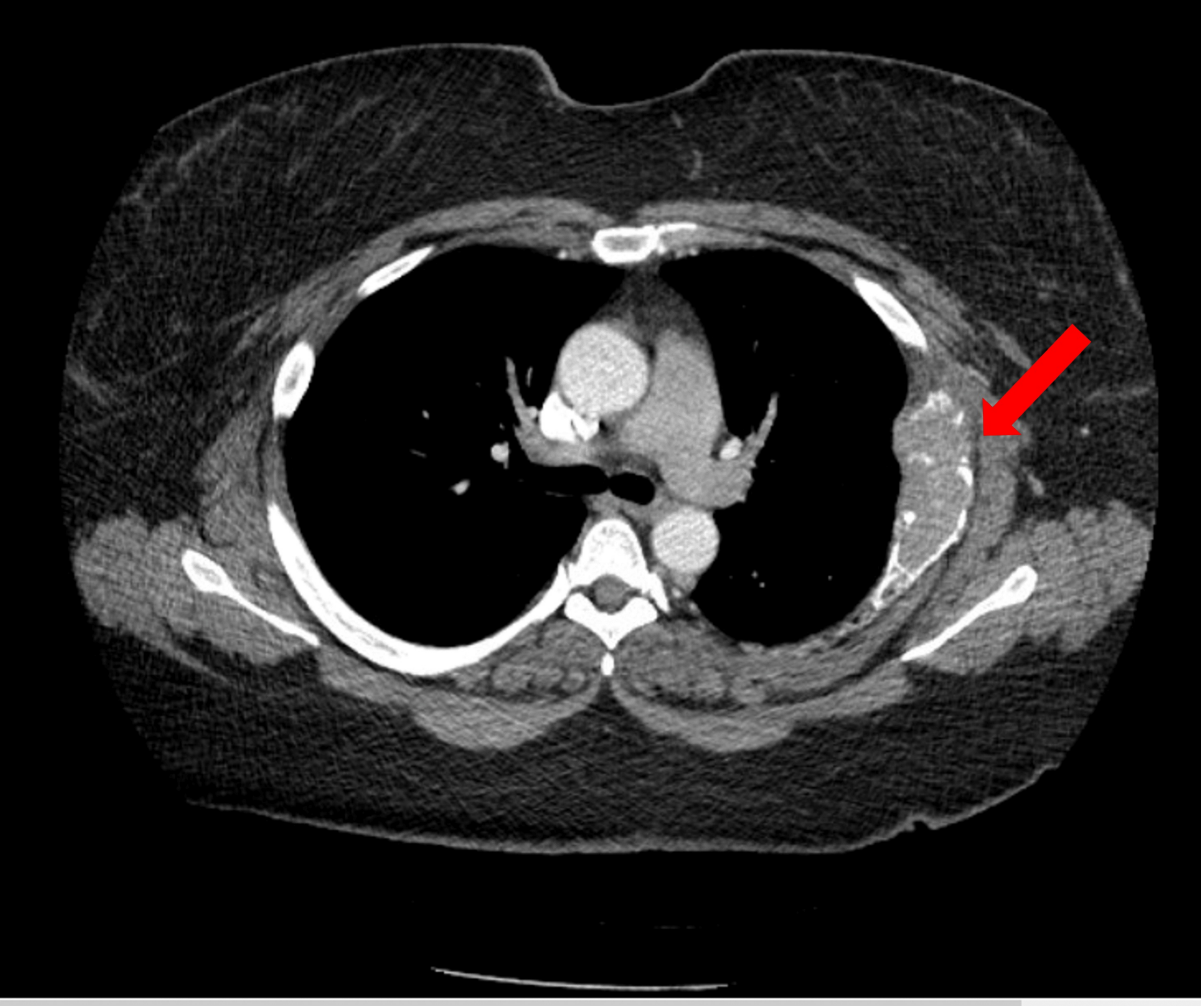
CT scan with contrast displaying an expansive osseous lesion of the left fifth rib
